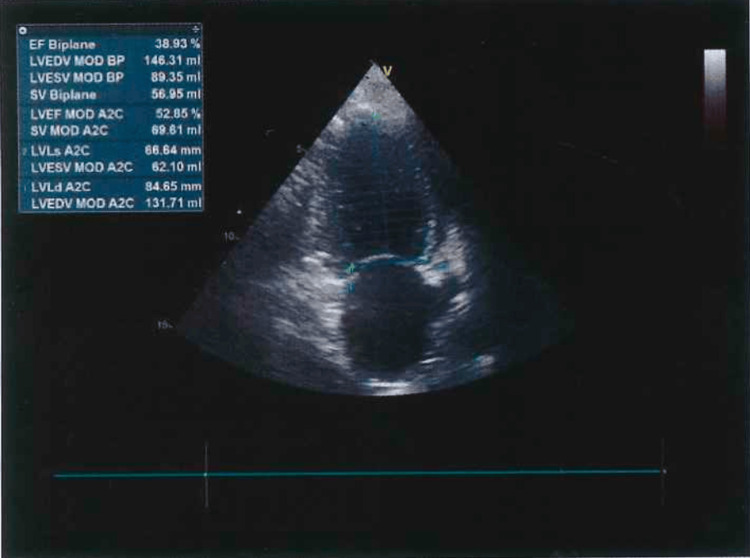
TTE at diagnosis TTE demonstrated reduced LVEF of 38% and PASP of 46 mmHg. LVEF, left ventricular ejection fraction; PASP, pulmonary artery systolic pressure; TTE, transthoracic echocardiography
